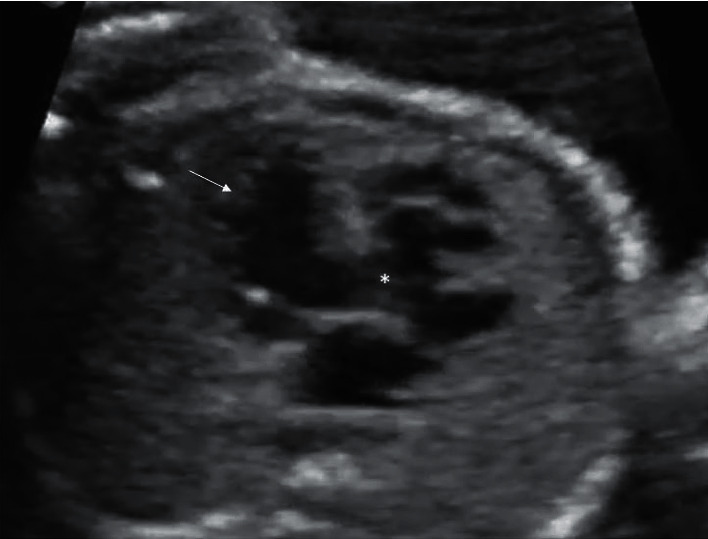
A 4-chamber view in a fetal echocardiogram showing a heavily trabeculated spongy looking LV suggestive of non-compaction (arrow). It also shows the Ebstein's anomaly and a VSD (^*∗*^) between the LV to the atrialized portion of the RV.
